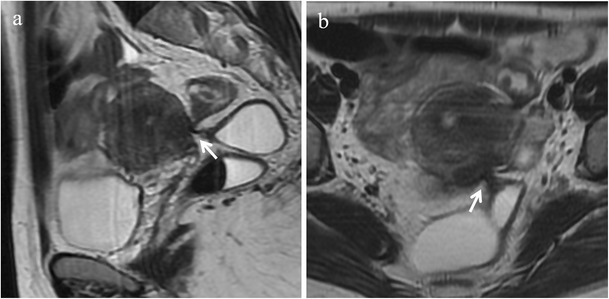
Adhesions in a 43-year-old woman. (a) Sagittal, and (b) oblique axial T2-weighted images display spiculated hypointense strands (white arrows) between the anterior surface of the sigma and the posterior uterine serosa with angulation of rectosigmoid colon
